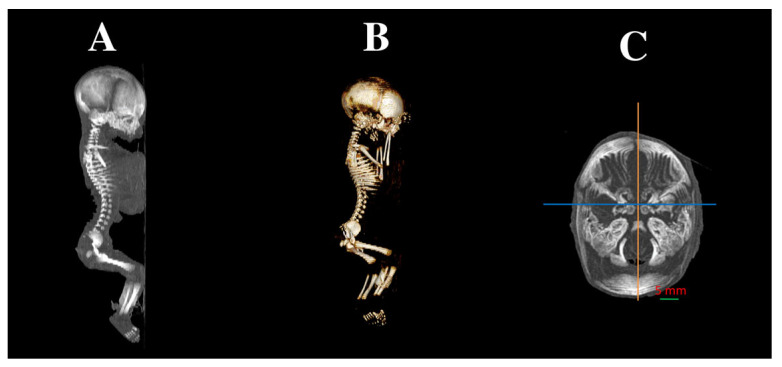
Computed tomography image of whole female human fetus at 25 weeks of gestation presented in sagittal view (A), accompanied by three-dimensional reconstruction in same projection (B), and cranial CT image in transverse plane (C).
